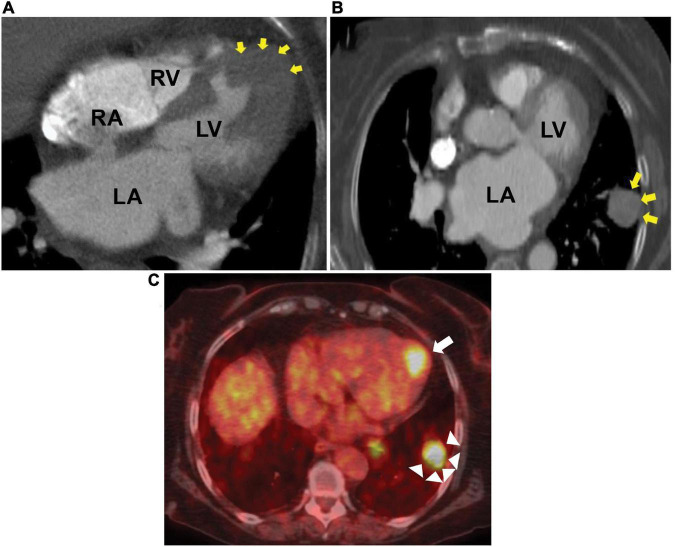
Chest CT and PET scan. Computed tomography (CT) showing metastatic melanoma involving the left ventricular apical wall (A), left lung (B), and positron emission tomography (PET) scan axial image (C) showing increased fluorodeoxyglucose uptake in the left ventricular apex (arrow) and left lung (arrow heads). LA, left atrium; LV, left ventricle; RA, right atrium; RV, right ventricle.
